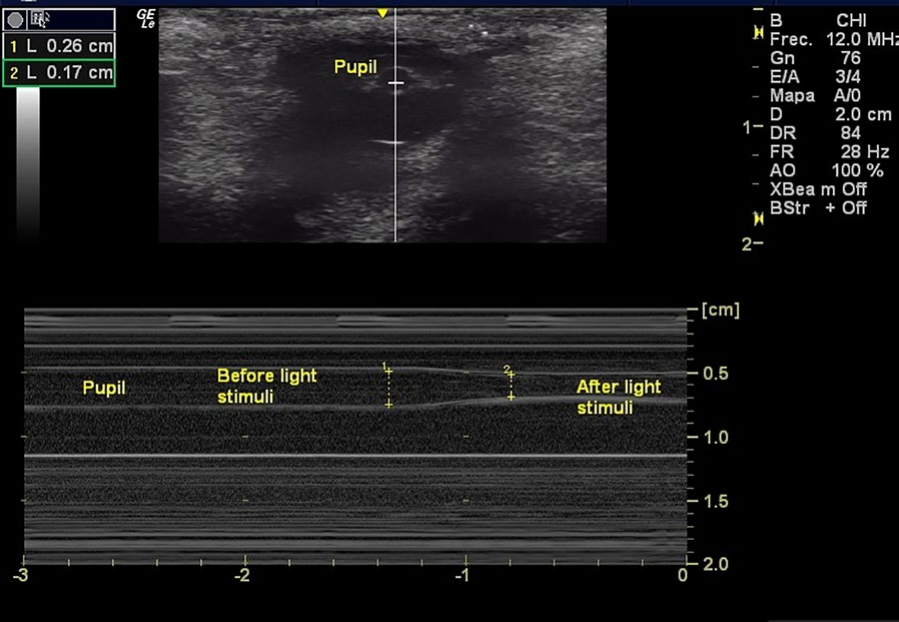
Ocular ultrasound on 2D (top) and M-mode (bottom) showing pupil before light stimuli and after light stimuli. Pupillary on M-mode tracing is highlighted by two white, horizontal, straight, parallel lines
